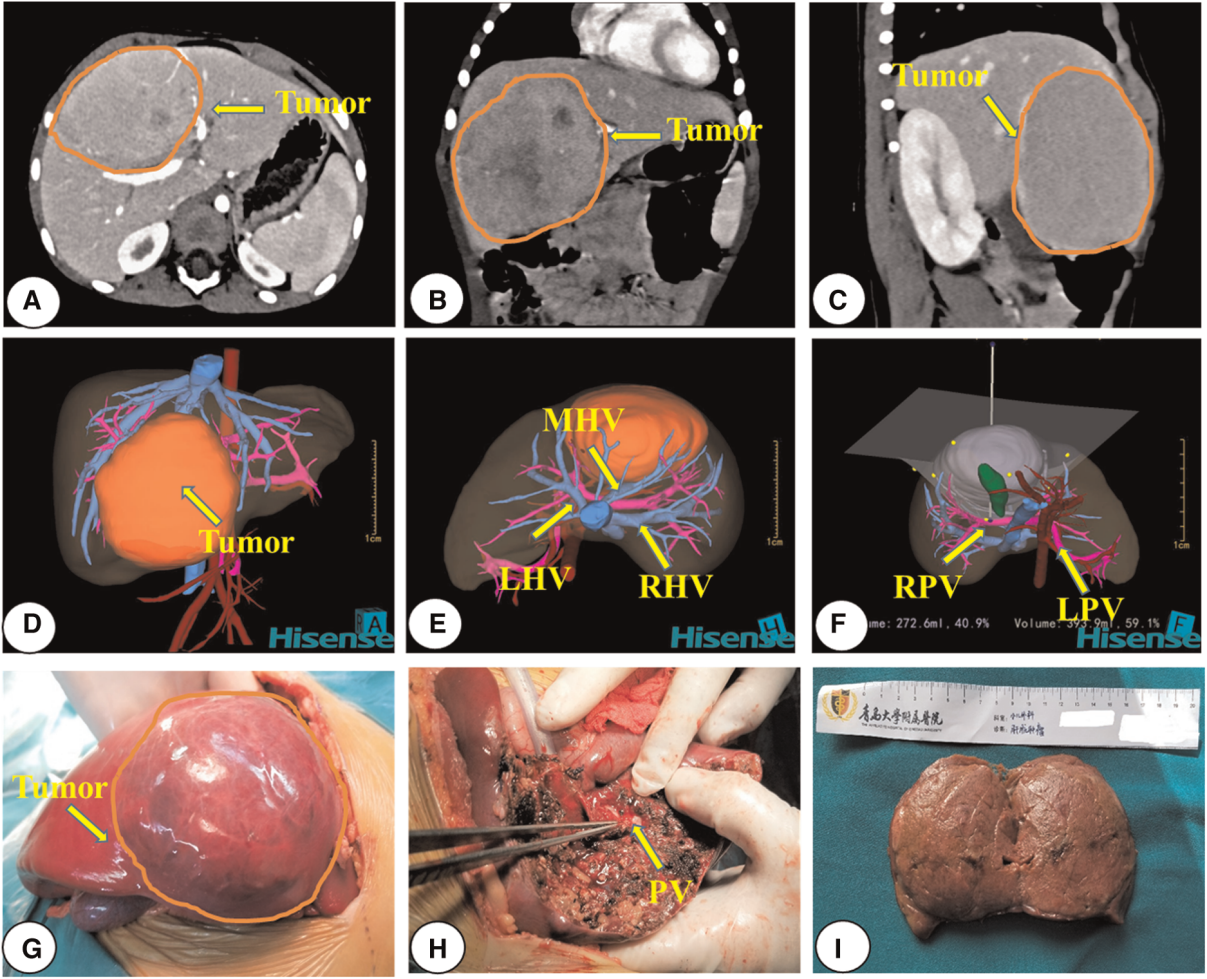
Representative preoperative assessment and surgical status of a patient with hepatoblastoma who underwent upfront surgery. (A–C) CT images; (D–F) 3D reconstruction images; (G–I) intraoperative observations. CT, computed tomography; 3D, three-dimensional; RPV, right portal vein; LPV, left portal vein; PV, portal vein; RHV, right hepatic vein; LHV, left hepatic vein; MHV, middle hepatic vein; AA, Abdominal Aorta.
